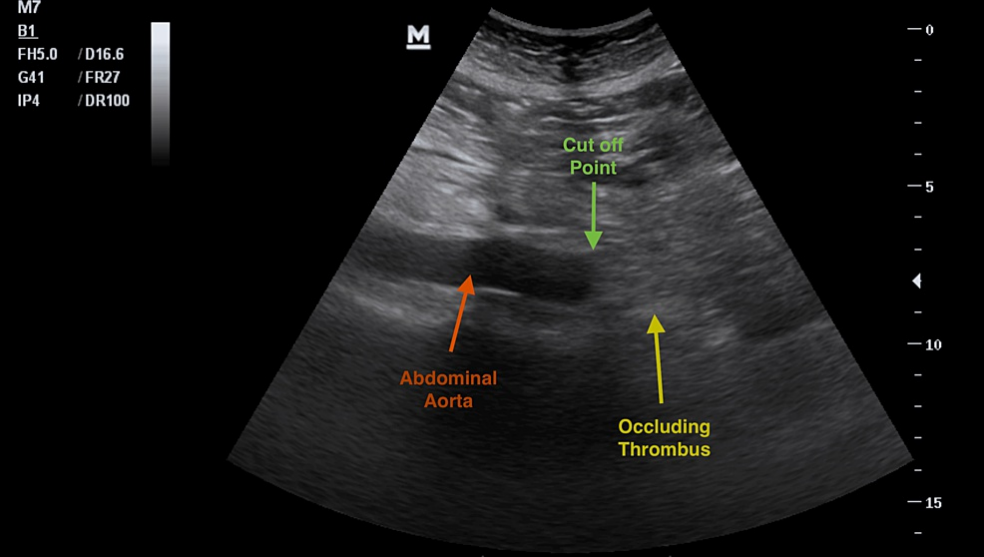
PoCUS - Longitudinal View of Abdominal Aorta PoCUS: point-of-care ultrasound
